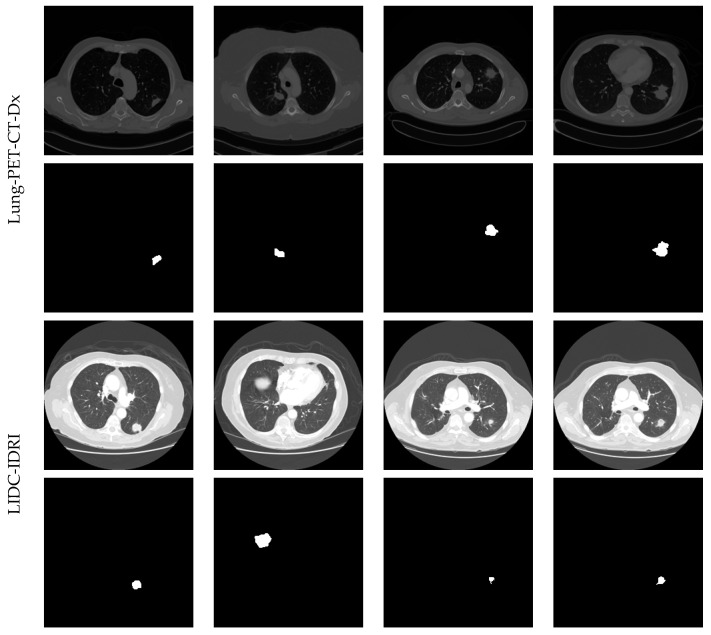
Segmentation of nodule from chosen images of Lung-PET-CT-Dx and LIDC-IDRI dataset.
